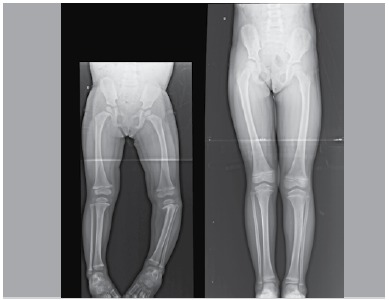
Panoramic x-ray before (left) and after (right) treatment with orthosis of a patient with Blount's disease to the left.
